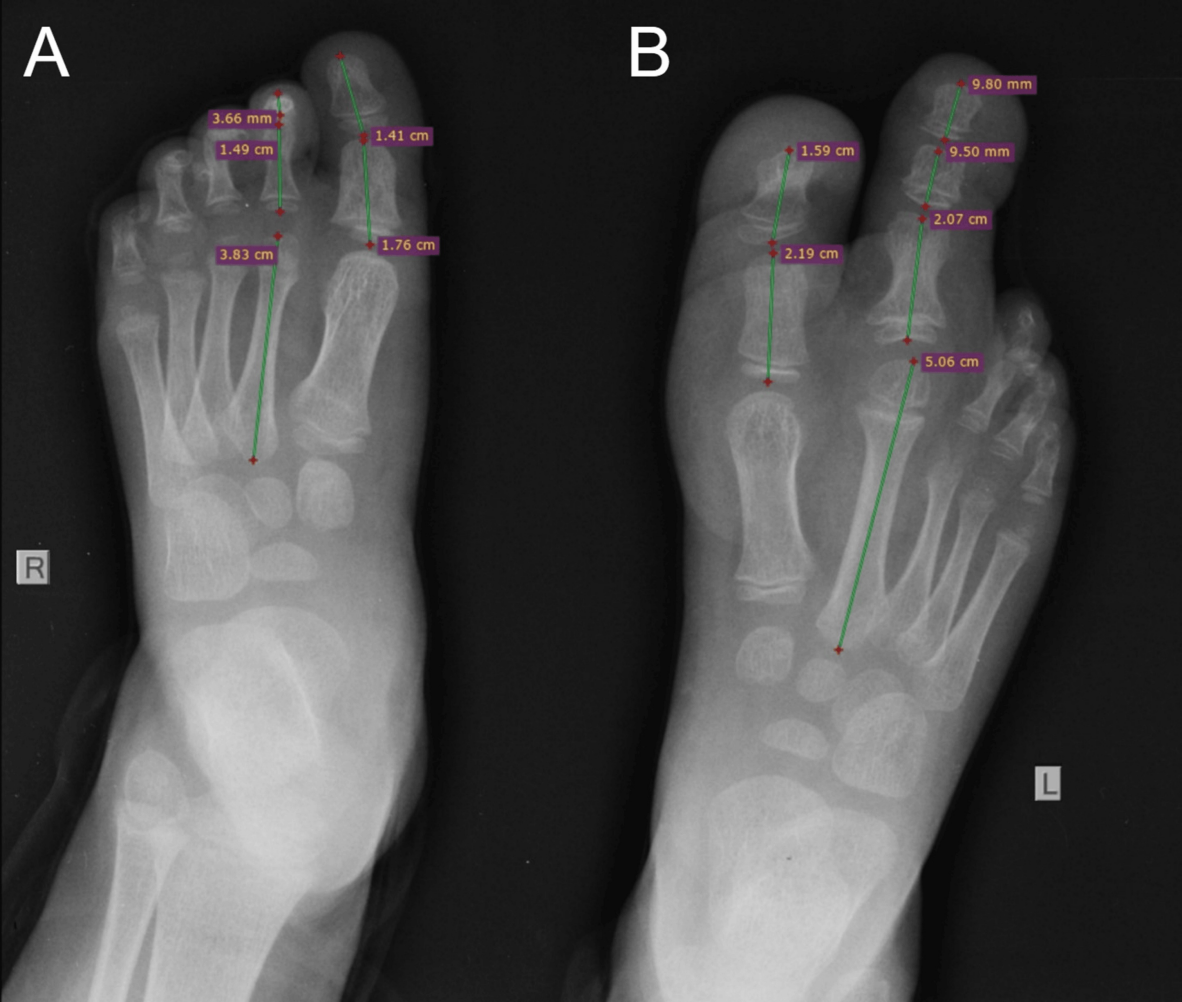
Antero-posterior (AP) X-ray at three years of age. Unaffected right foot (A) compared with the left macrodactyly one (B).
